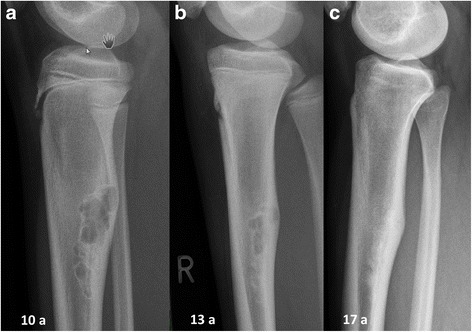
(a) A girl aged 10 years at the first presentation. Lateral radiographs of the right proximal tibia showing a typical NOF in stage B: distant from the epiphysis, polycyclic shaped and with clearly sclerotic borders. The cortex is protruding like the shape of an hourglass. (b) Three years later typical ossification beginning at the diaphysis is evident, representing a stage C lesion. (c) Complete homogeneous sclerosis of the lesion at the patient age of 17 years
